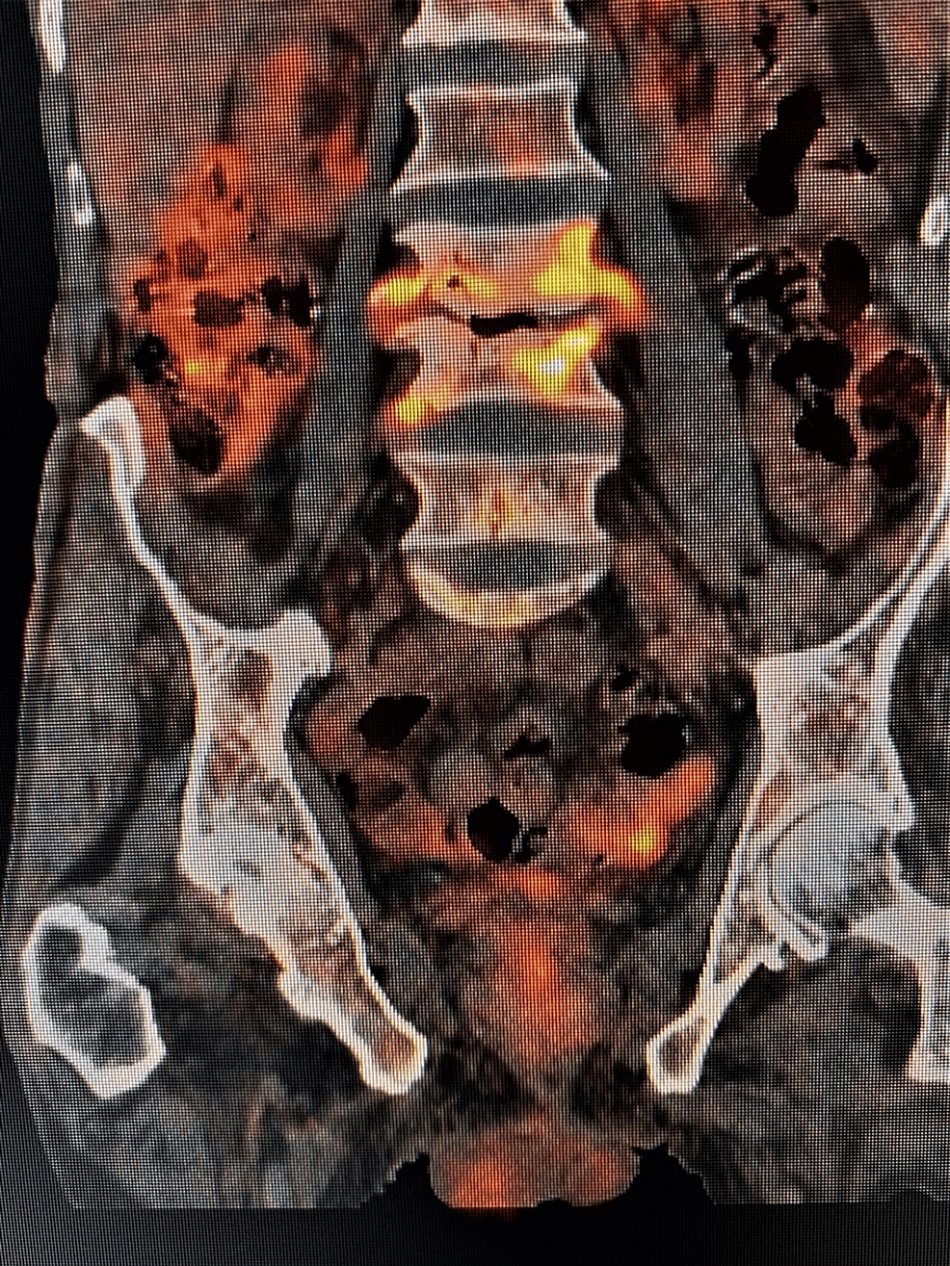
FDG PET/CT of the same patient as above after six months showing a decrease in FDG uptake in L3 - L4 intervertebral disc (SUVmax = 4.1) FDG - fluorodeoxyglucose, PET - positron emission tomography, SUVmax - maximum standardized uptake value
